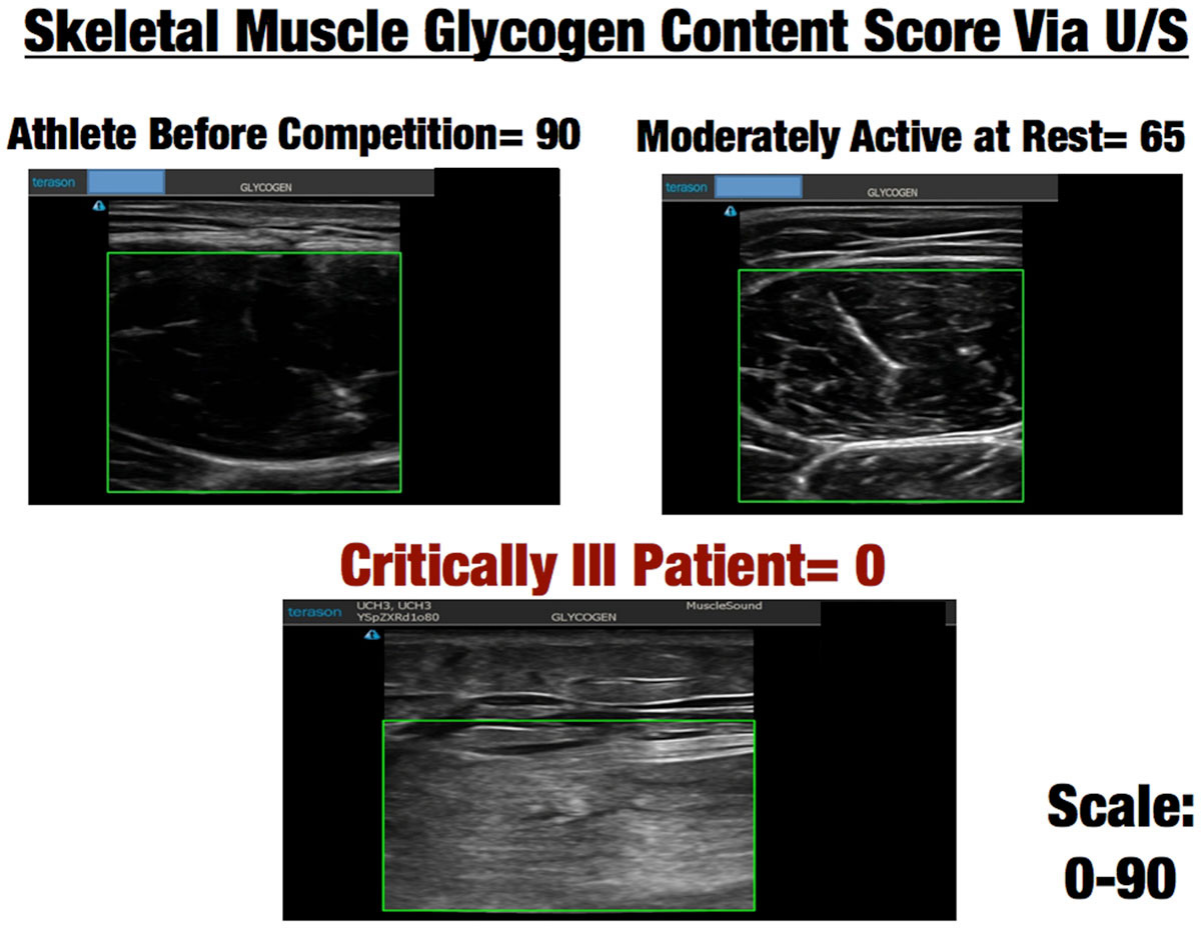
Skeletal muscle glycogen content score via ultrasound.
